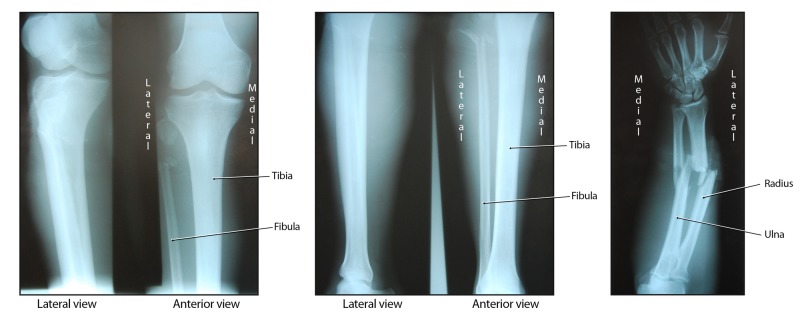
Radiographic images of the patient's polytraumatic presentation.
